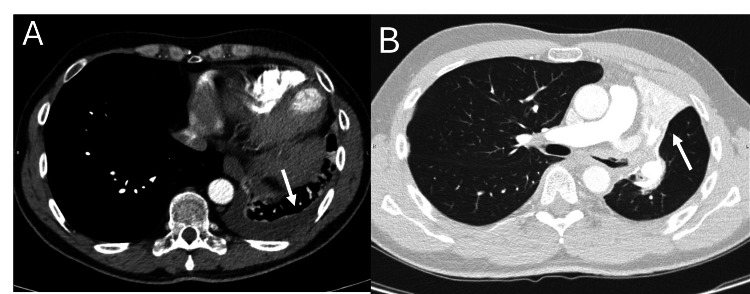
Computed tomography of the chest at first presentation. (A) Computed tomography of the chest shows left-sided pleural effusion (arrow). (B) The lung window shows complete atelectasis of the left upper lobe and lingula (arrow) due to obstruction of the left upper lobe bronchus.
